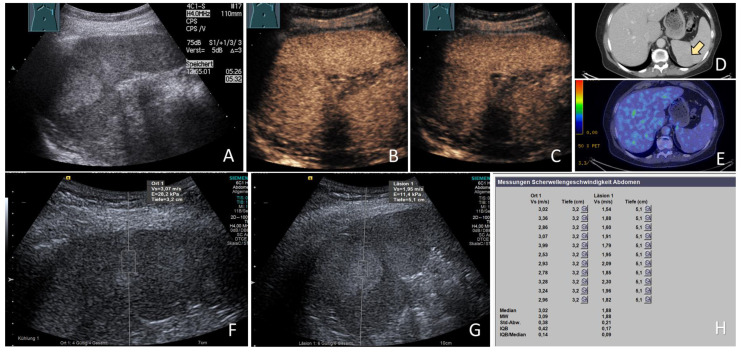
Benign focal splenic lesion (bFSL). 69-year-old asymptomatic female patient with an incidental finding of an FSL; (A) B-mode US showing a large echogenic splenic mass, (B) on CEUS, the FSL showed an arterial phase isoenhancement with (C) mild washout in the late phase after 180 s, (D) CT scan of the same FSL (arrow) (courtesy of A. Mahnken, Department of Radiology, University Hospital Marburg), (E) PET-CT showing no tracer accumulation within the lesion (courtesy of M. Luster, Department of Nuclear Medicine, University Hospital Marburg), (F) US elastography image showing an ARFI measurement of 3.07 m/s within the splenic parenchyma, (G) US elastography image showing an ARFI measurement of 1.95 m/s within the lesion, and (H) the final ARFI report of the splenic parenchyma (Ort 1) showing a mean ARFI velocity (MW) of 3.09 m/s and of the lesion (Läsion 1) showing a mean ARFI velocity (MW) of 1.88 m/s. A diagnosis of benign vascular lesion (hemangioma) was established and the lesion remained unchanged on follow up at 10 months.
